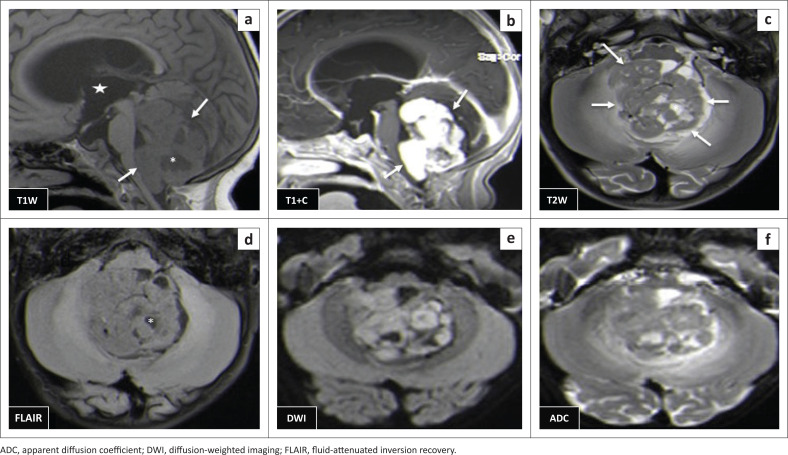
(a–f) Medulloblastoma, predominantly centred in the fourth ventricle. The mass is predominantly solid (arrows) with tiny cystic areas (*) and avid enhancement of the solid areas (b). Two images (c, d) show T2 and FLAIR low signal of dense hypercellular solid areas and fluid restriction on DWI and ADC map. Supratentorial hydrocephalus is also seen (star).
